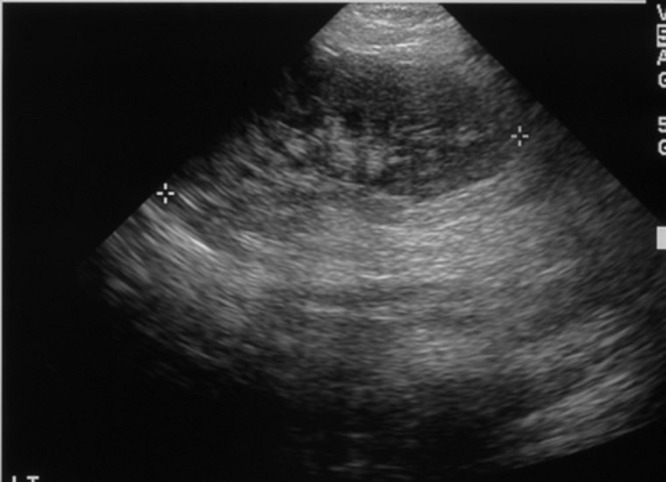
Figure 2: Renal ultrasound approximately one year following reduction of prolapse, showing complete resolution of left hydronephrosis. Again, right hydronephrosis had also resolved.
